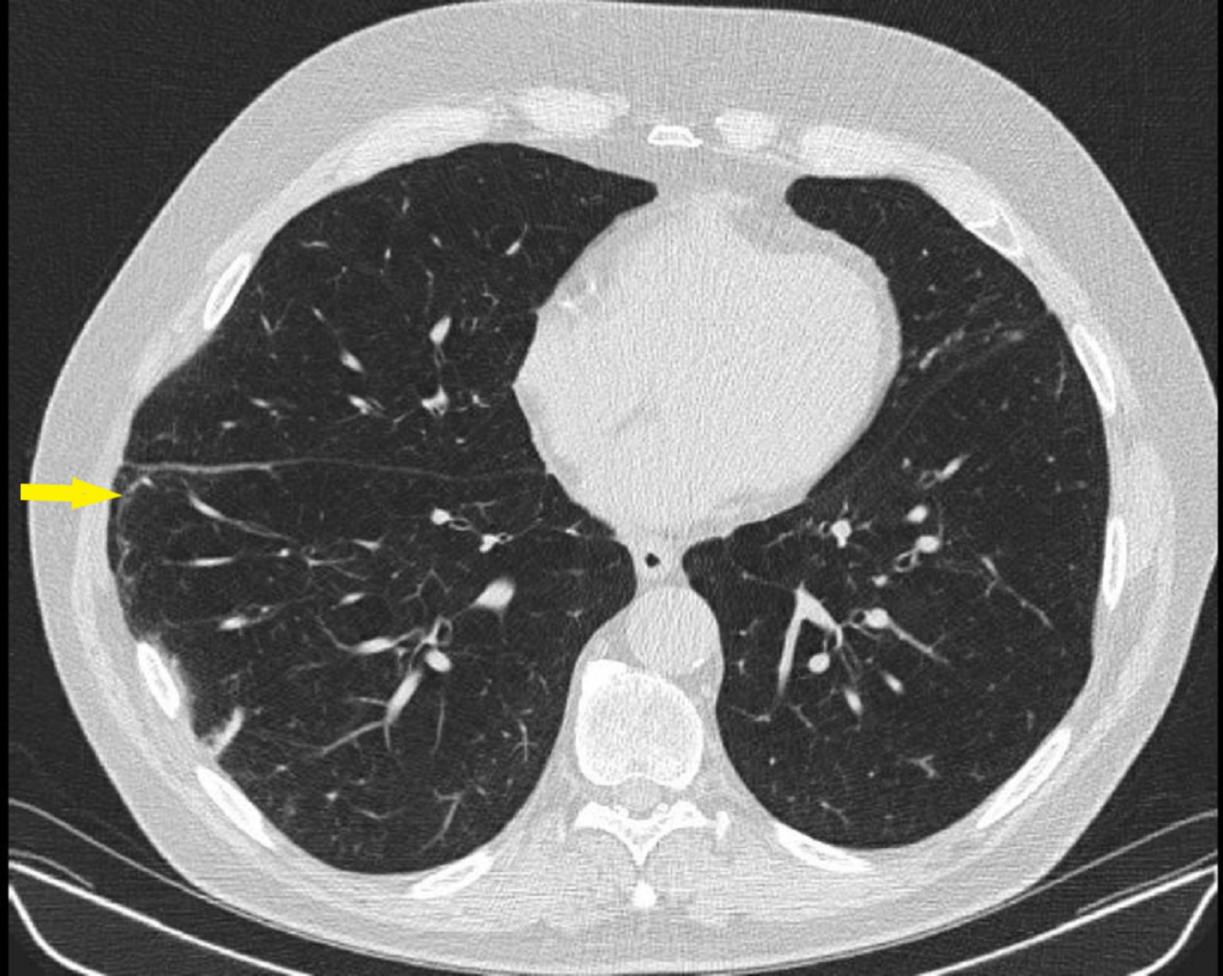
CT chest coronal view showing persistent lung herniation and splaying of ribs (yellow arrow) CT: computed tomography
